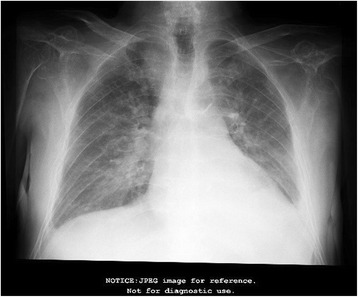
Chest X-ray on day 12. Pulmonary edema plus new costal fractures
